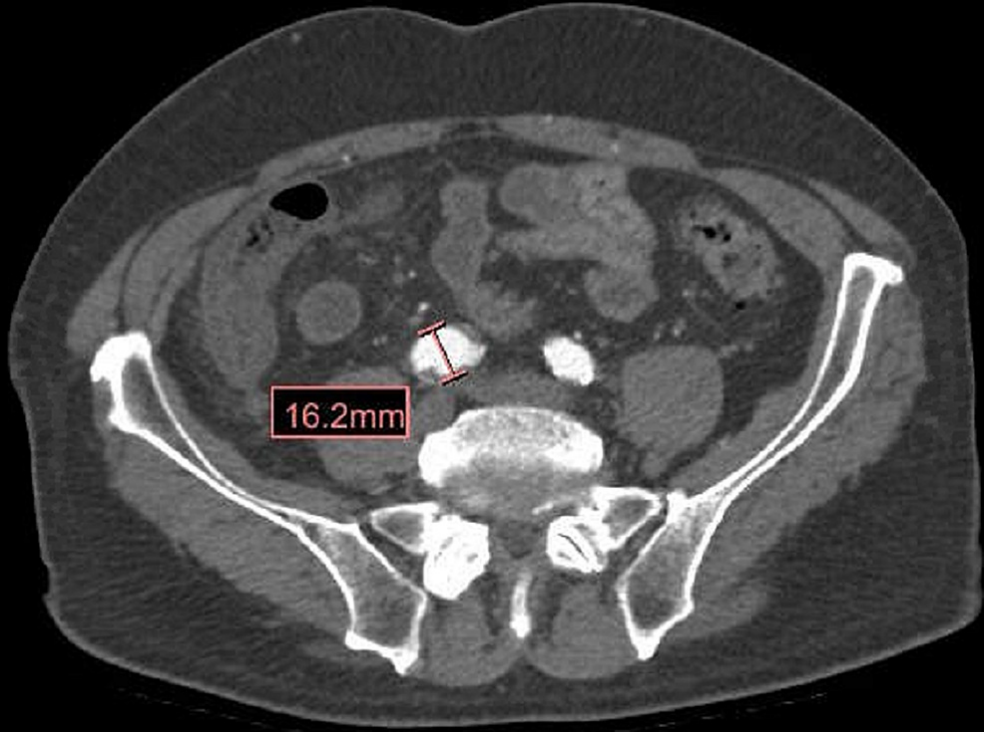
CT angiogram aorta demonstrating right common iliac artery aneurysm measuring 1.6 cm
